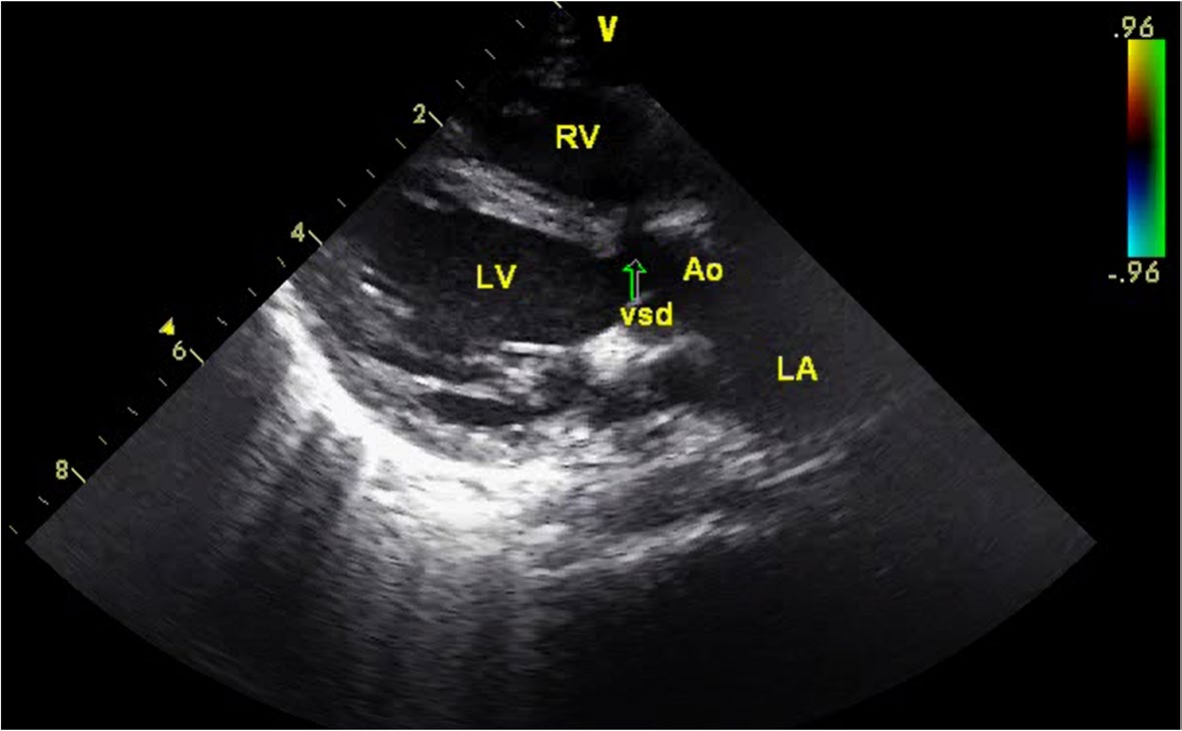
VSD echocardiographic view
